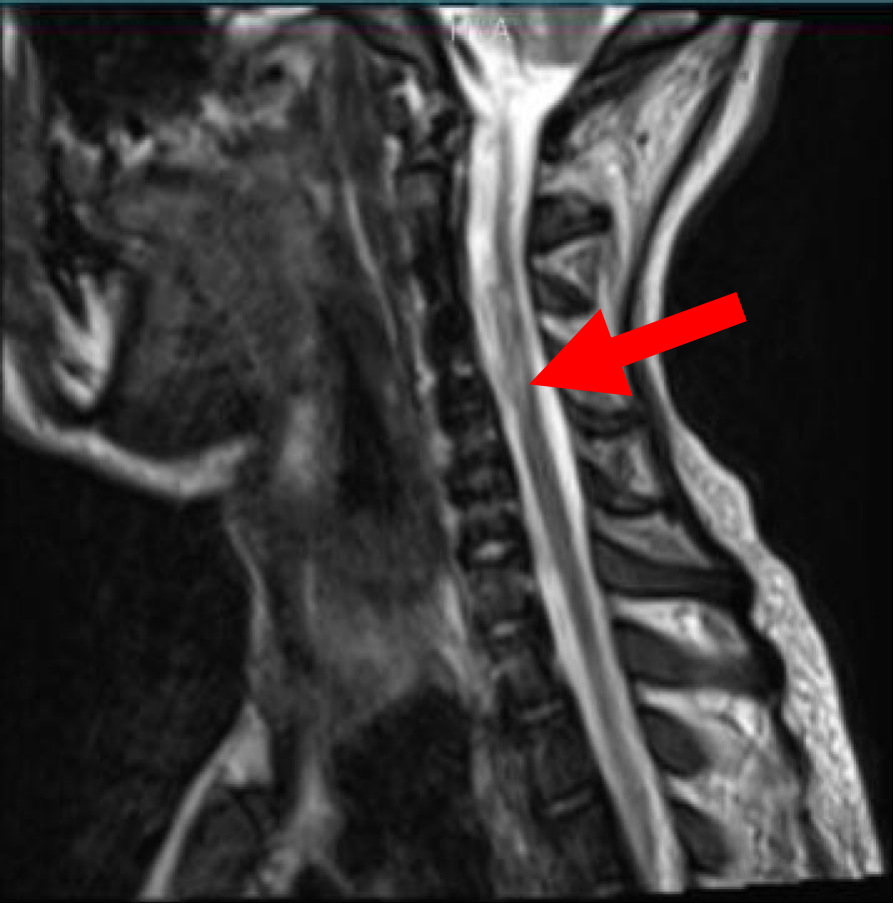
Repeat MRI T2_tse sagittal images, 1 month after starting IVIG and corticosteroid therapy
